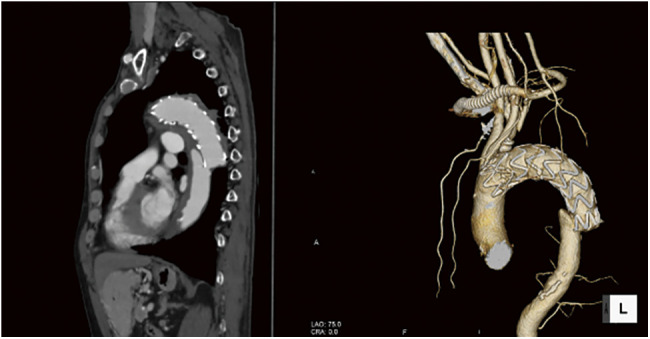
Fig. 2 CT performed at 3 years after TEVAR shows a significant deformity of the descending aorta. CT: computed tomography; TEVAR: thoracic endovascular aortic repair
